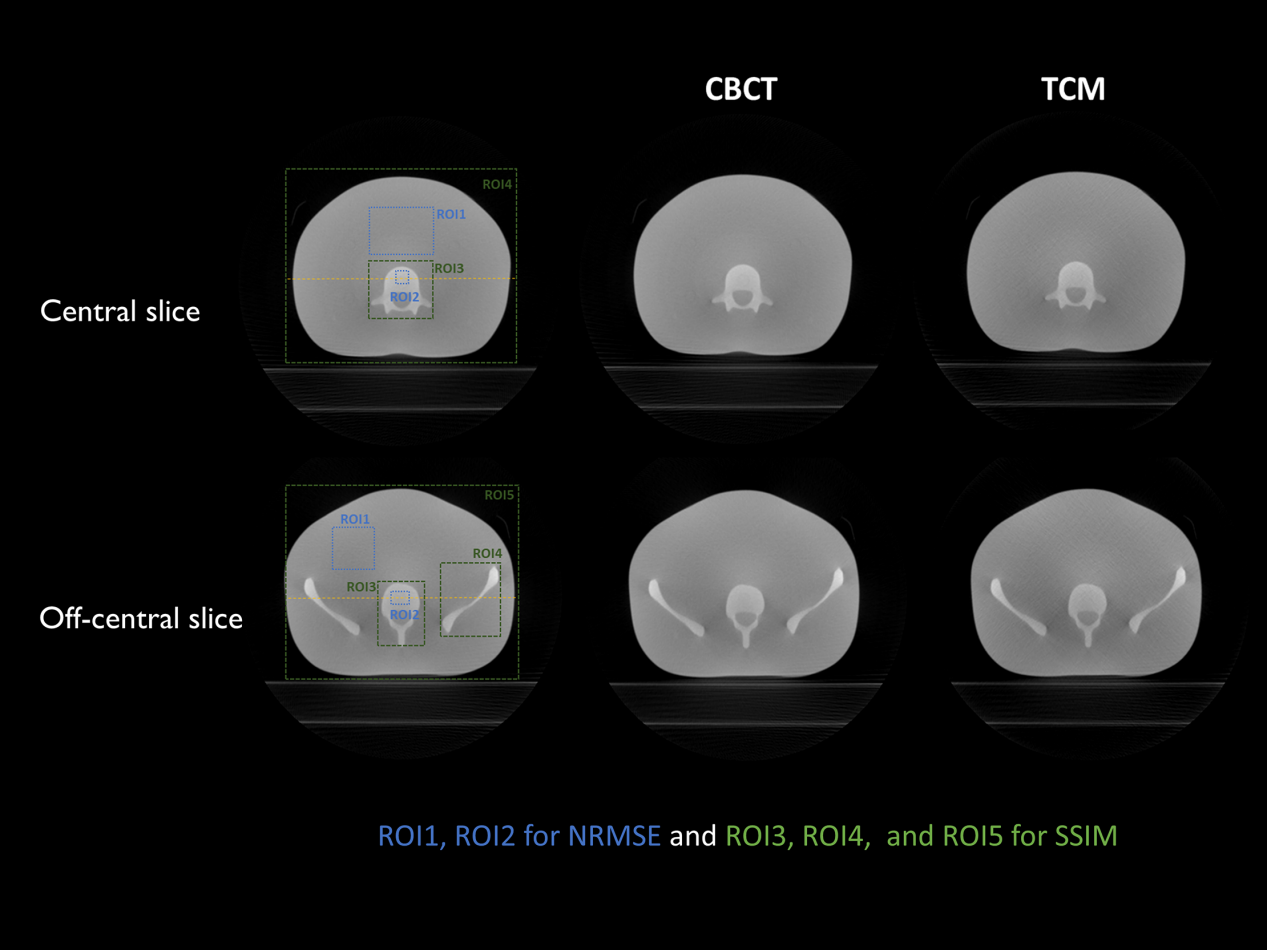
Reconstructed images of the ATOM’s abdominal region using conventional CBCT and TCM methods. XVI images are obtained via central and off-center slices.
